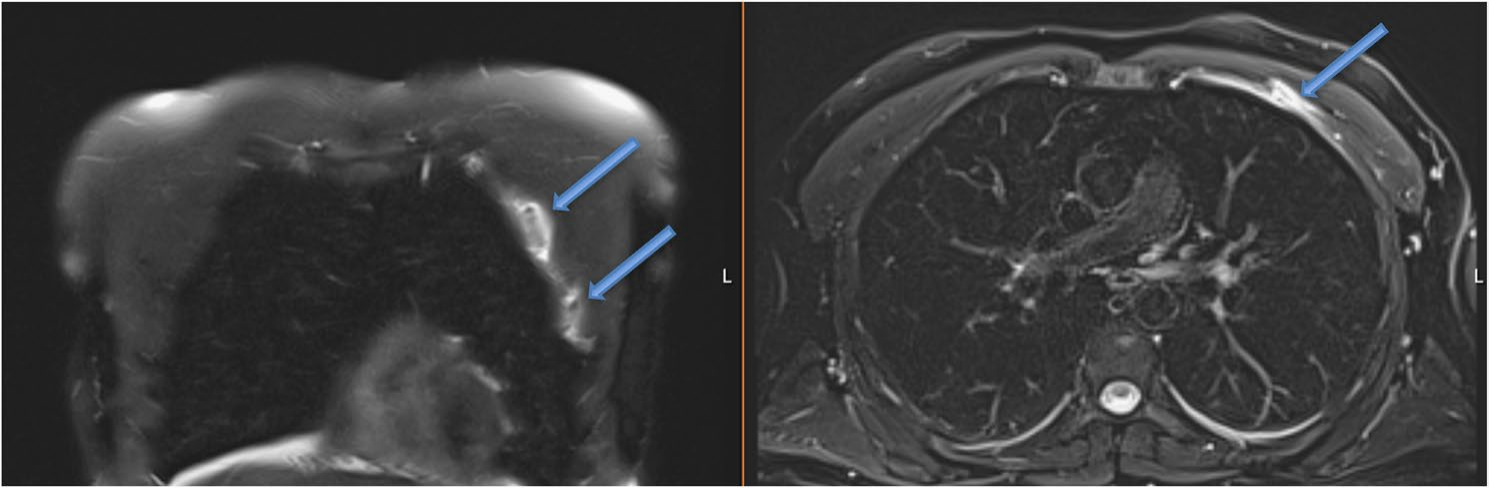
Coronal STIR demonstrates adjacent rib edema. Axial PD-FS MRI shows a partial pectoralis minor tear at the costal origin (arrows) near the 3rd–4th costochondral junction
